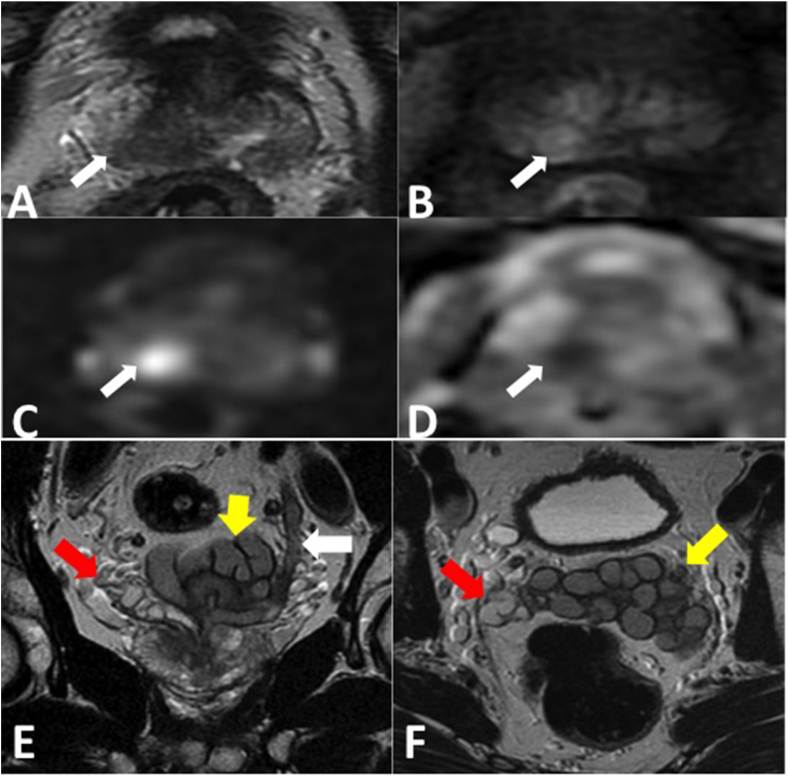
A-D: Multi-parametric MRI. Axial T2w (A), dynamic contrast enhanced (B) DWI (C) and ADC map (D) show a hypointense circumscribed area of low T2 signal intensity in the right medial basal peripheral zone (arrow) which displays focal early asymmetrical enhancement and is high signal on the DWI with corresponding low signal intensity on the ADC. This is scored as a PI-RADS 4/5 lesion as the size of the abnormality is less than 1.5 cm, stage T2. Targeted biopsy revealed Gleason 3 + 4. E& F: Coronal T2w(E) and axial T2w(F) of the prostate and seminal vesicles show the ectopic insertion of the left ureter (white arrow) into the left seminal vesicle. The left seminal vesicle (yellow arrow) is asymmetrically enlarged compared to the normal sized right seminal vesicle (red arrow). (For interpretation of the references to colour in this figure legend, the reader is referred to the Web version of this article.)
